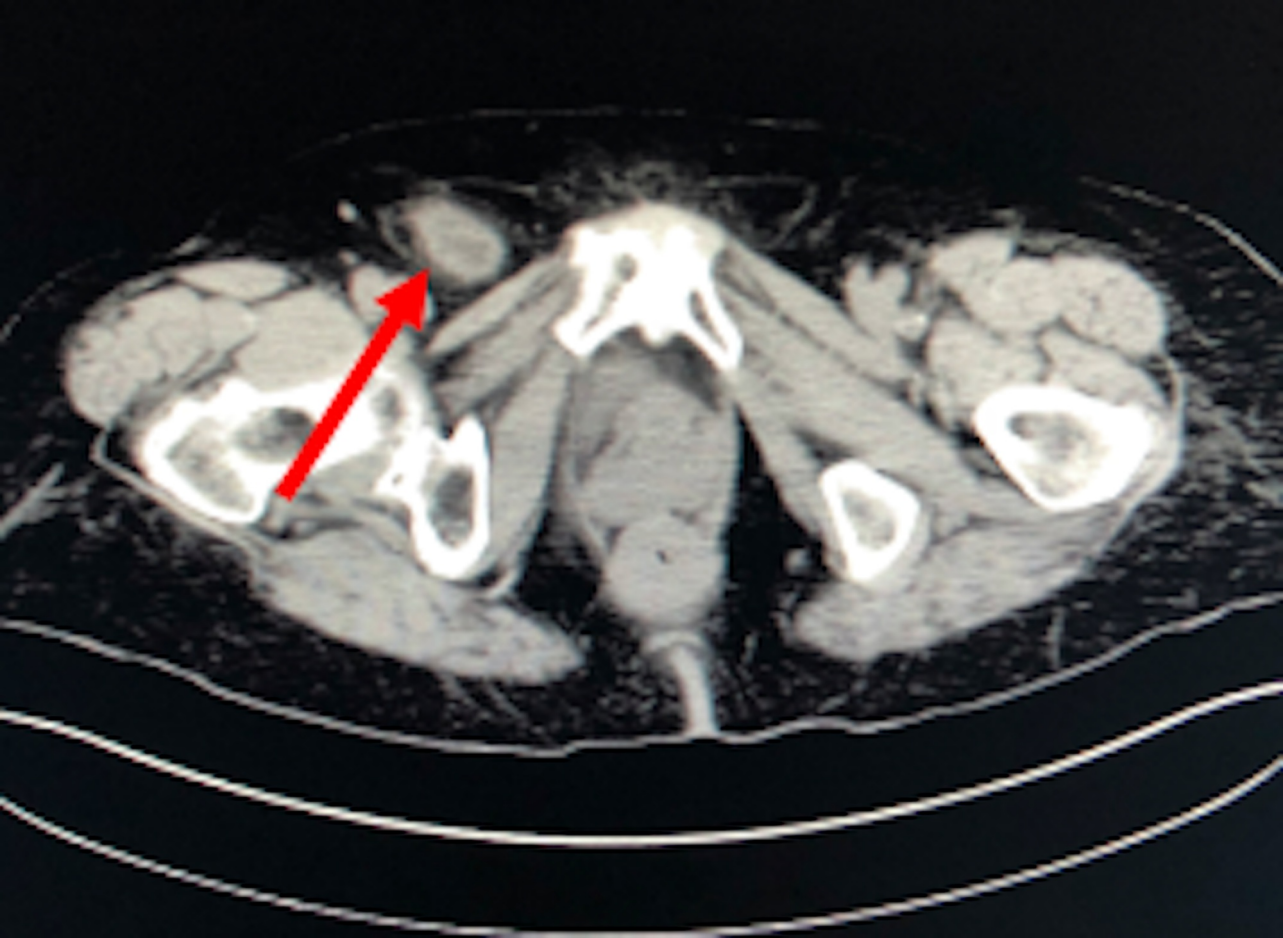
CT abdomen/pelvis axial view: the arrow indicating herniated bladder diverticulum
